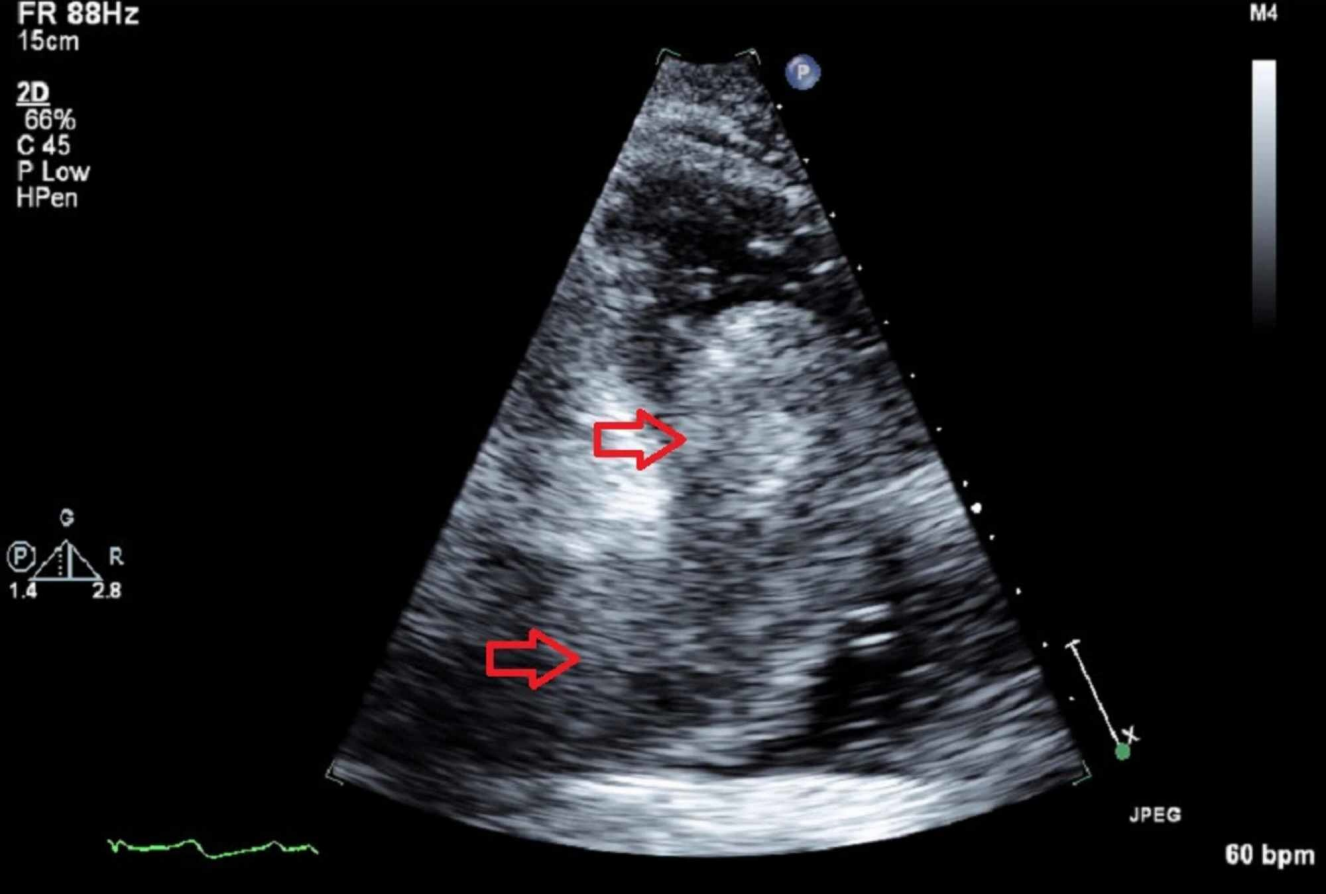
Apical two-chamber transthoracic echocardiogram view shows the mass in the right side of the heart. The arrow at the bottom shows the mass when entering the right atrium.
